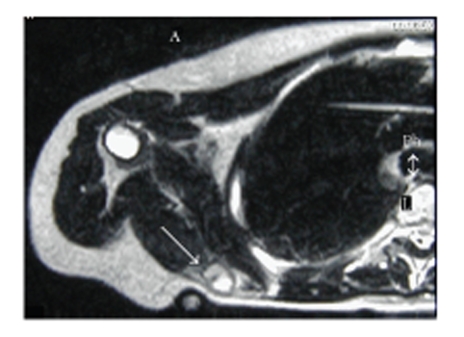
MRI of the right scapula showing a decrease in size
